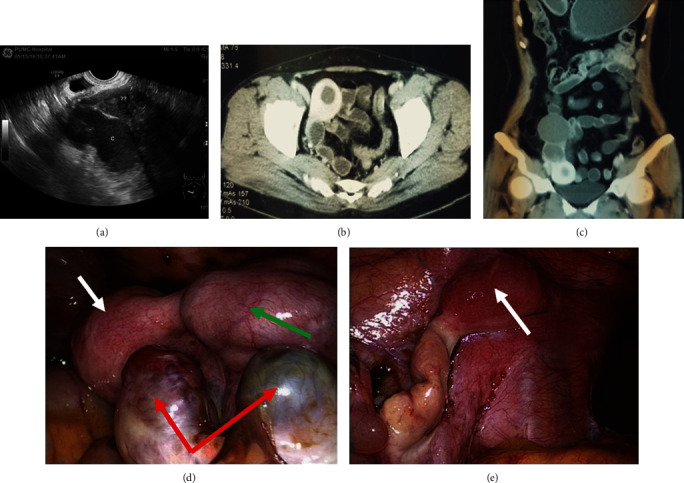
(a) Preoperative ultrasonography: a hypoechoic lesion of 4.2 × 3.9 × 3.1 cm was found in the right adnexal region, in which there was an isoecho lesion of 1.5 × 0.7 cm. Circumferential blood flow signal was observed by CDFI. An anechoic cyst of 7.2 × 3.9 × 3.8 cm was also seen outside the hypoechoic lesion mentioned above. (b, c) MRI shows bilateral residual uterus with the endometrium in the right, and a segmental low-signal cystic lesion is located on the upper right of the right uterus. The solid nodule on the left was in the inner wall of the ileum. (d, e) Laparoscopic findings. Bilateral residual uteri of maximum diameter (d) 5-6 cm on the right and (e) 2–3 cm on the left, fallopian tubes, and right ovarian chocolate cysts. The white arrow points to the uteri. The red arrow points to the ovarian cyst. The green arrow points to the swollen fallopian tube with hematocele.
